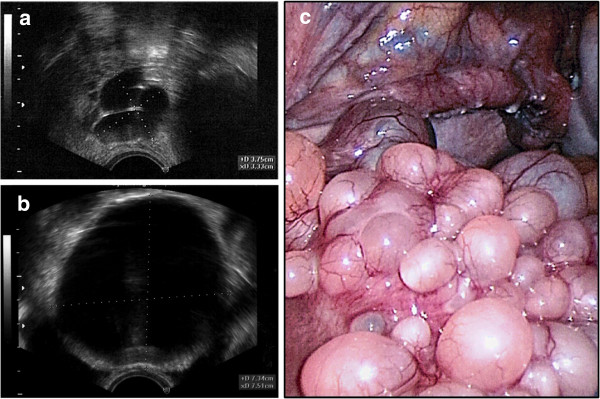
Clinical presentation. Transvaginal ultrasound showed cystic formations in the areas of the right fallopian tube (a) and fundus uteri (b). The maximum diameter of the cysts was 7.5 cm. Laparoscopic presentation of the cystic mass (c); uterus (front) with right tube and right abdominal wall (background).
